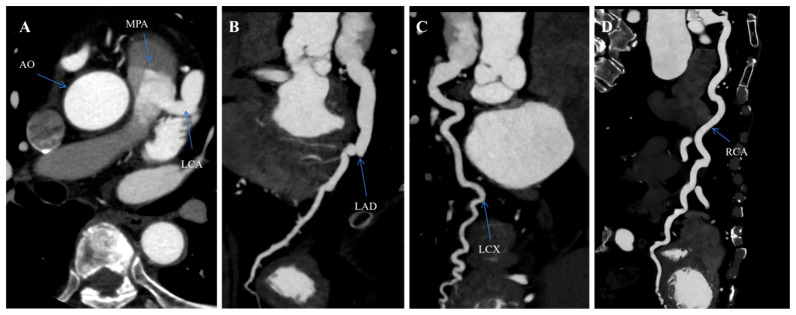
A 66-year-old woman presenting with intermittent chest pain and upper back pain for three months. (A) Axial coronary CTA image demonstrates anomalous origin of the left coronary artery (LCA; arrow) from the main pulmonary artery (MPA; arrow), with high-density contrast medium shunting into the MPA. Curved planar reformation (CPR) images clearly delineate the full course of the anomalous vessel from its anomalous ostium in the MPA, coursing along the expected left atrioventricular groove, giving rise to the LAD ((B); arrow) and LCX ((C); arrow). The proximal LAD shows marked luminal dilatation. (D) The right coronary artery (RCA; arrow) is significantly dilated and tortuous. The high spatial resolution of CPR enables precise evaluation of the anomalous ostial location, vessel caliber, and collateral development—information that is valuable for anatomic understanding and communication among the multidisciplinary team in adult ALCAPA patients.
